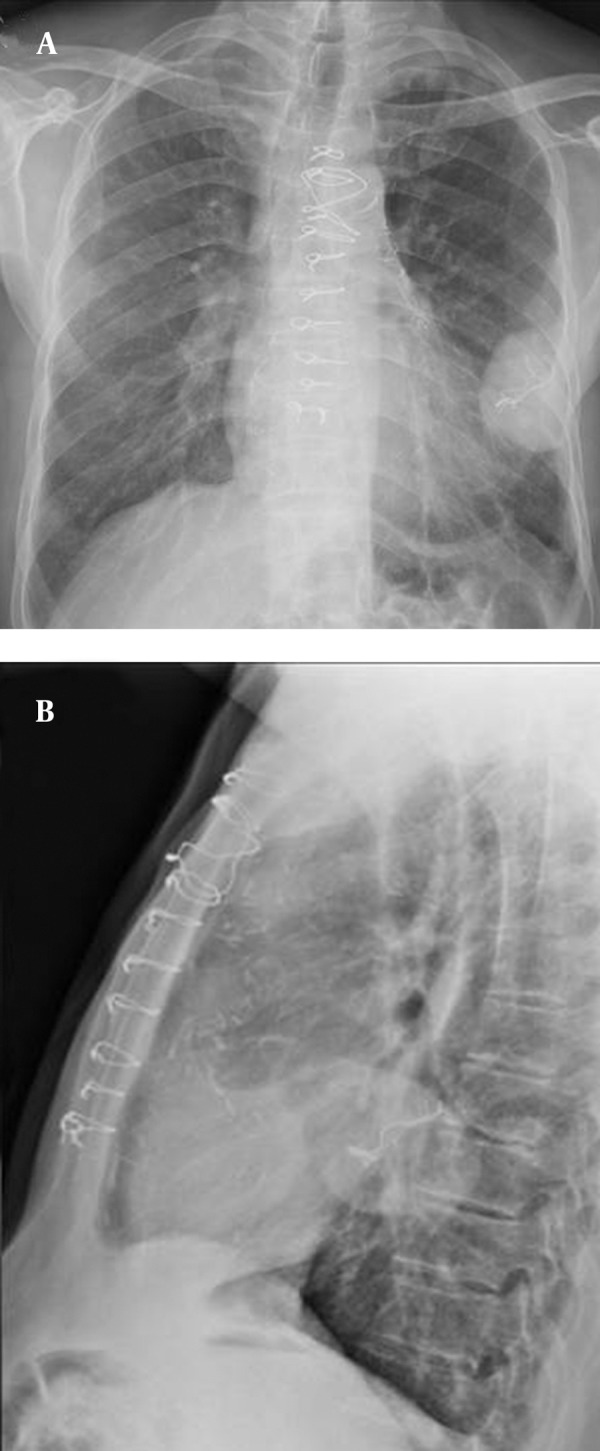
A) Posteroanterior and B) Lateral chest radiograph revealed a well-marginated homogeneous opacity in the middle-lower zone of the left hemithorax with striped appearance in the center
